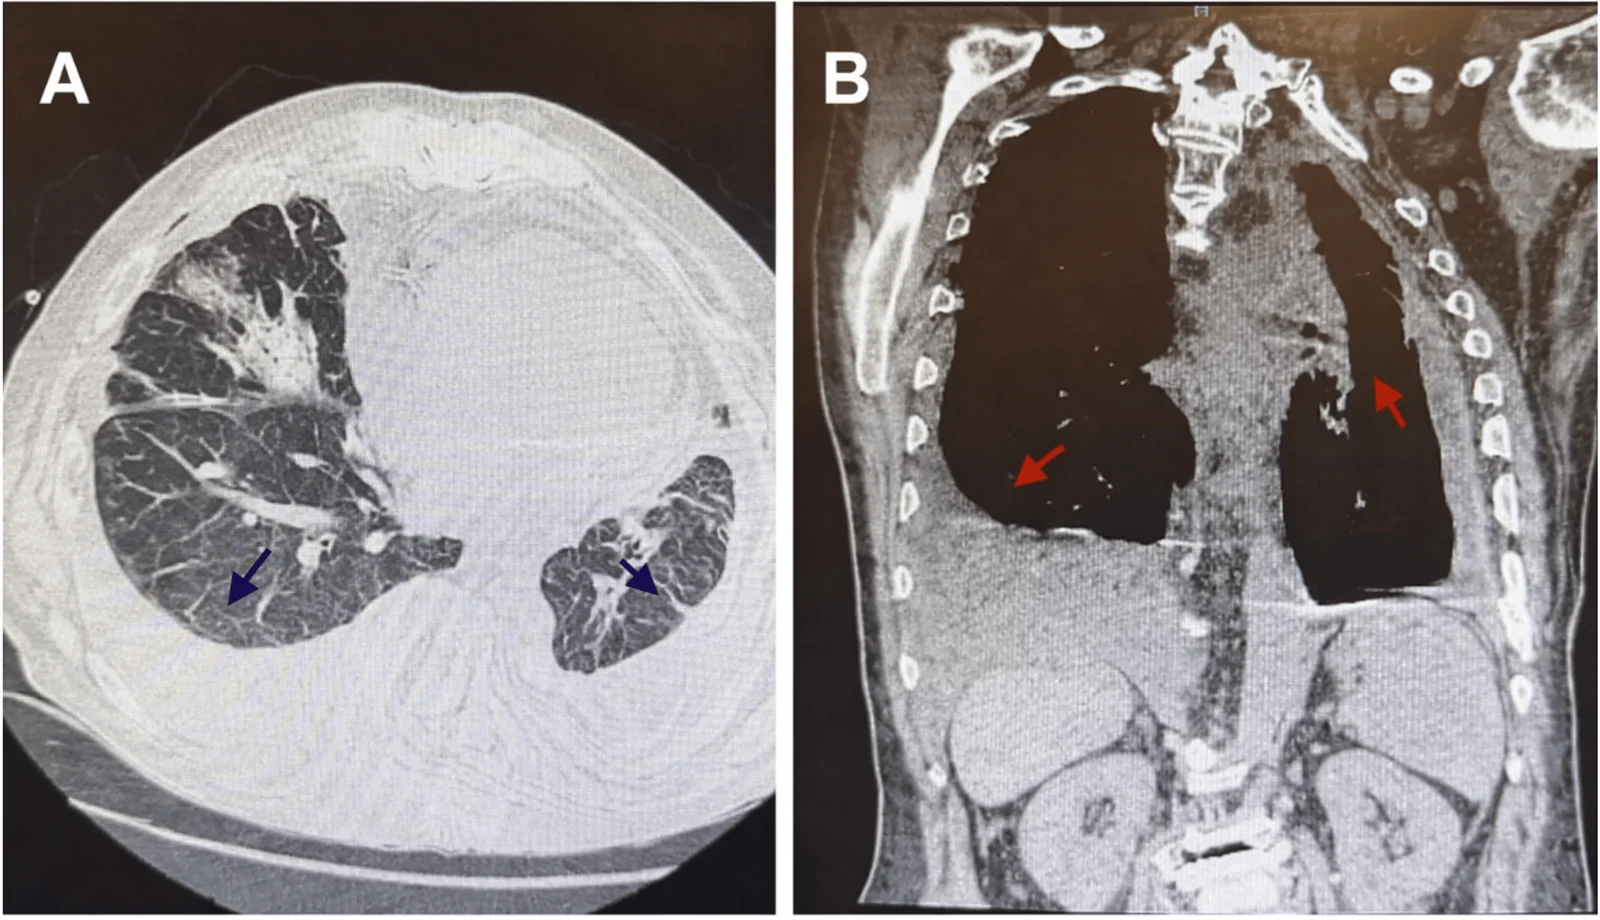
CT angiography of the chest obtained on hospital Day 1 demonstrating no acute pulmonary embolism (A) Axial image showing moderate bilateral pleural effusions, greater on the right, with adjacent compressive atelectatic change and pulmonary edema. (B) Coronal image demonstrating similar findings. Exclusion of pulmonary embolism redirected evaluation toward the pleural process, which was subsequently confirmed to represent Pasteurella multocida empyema.
